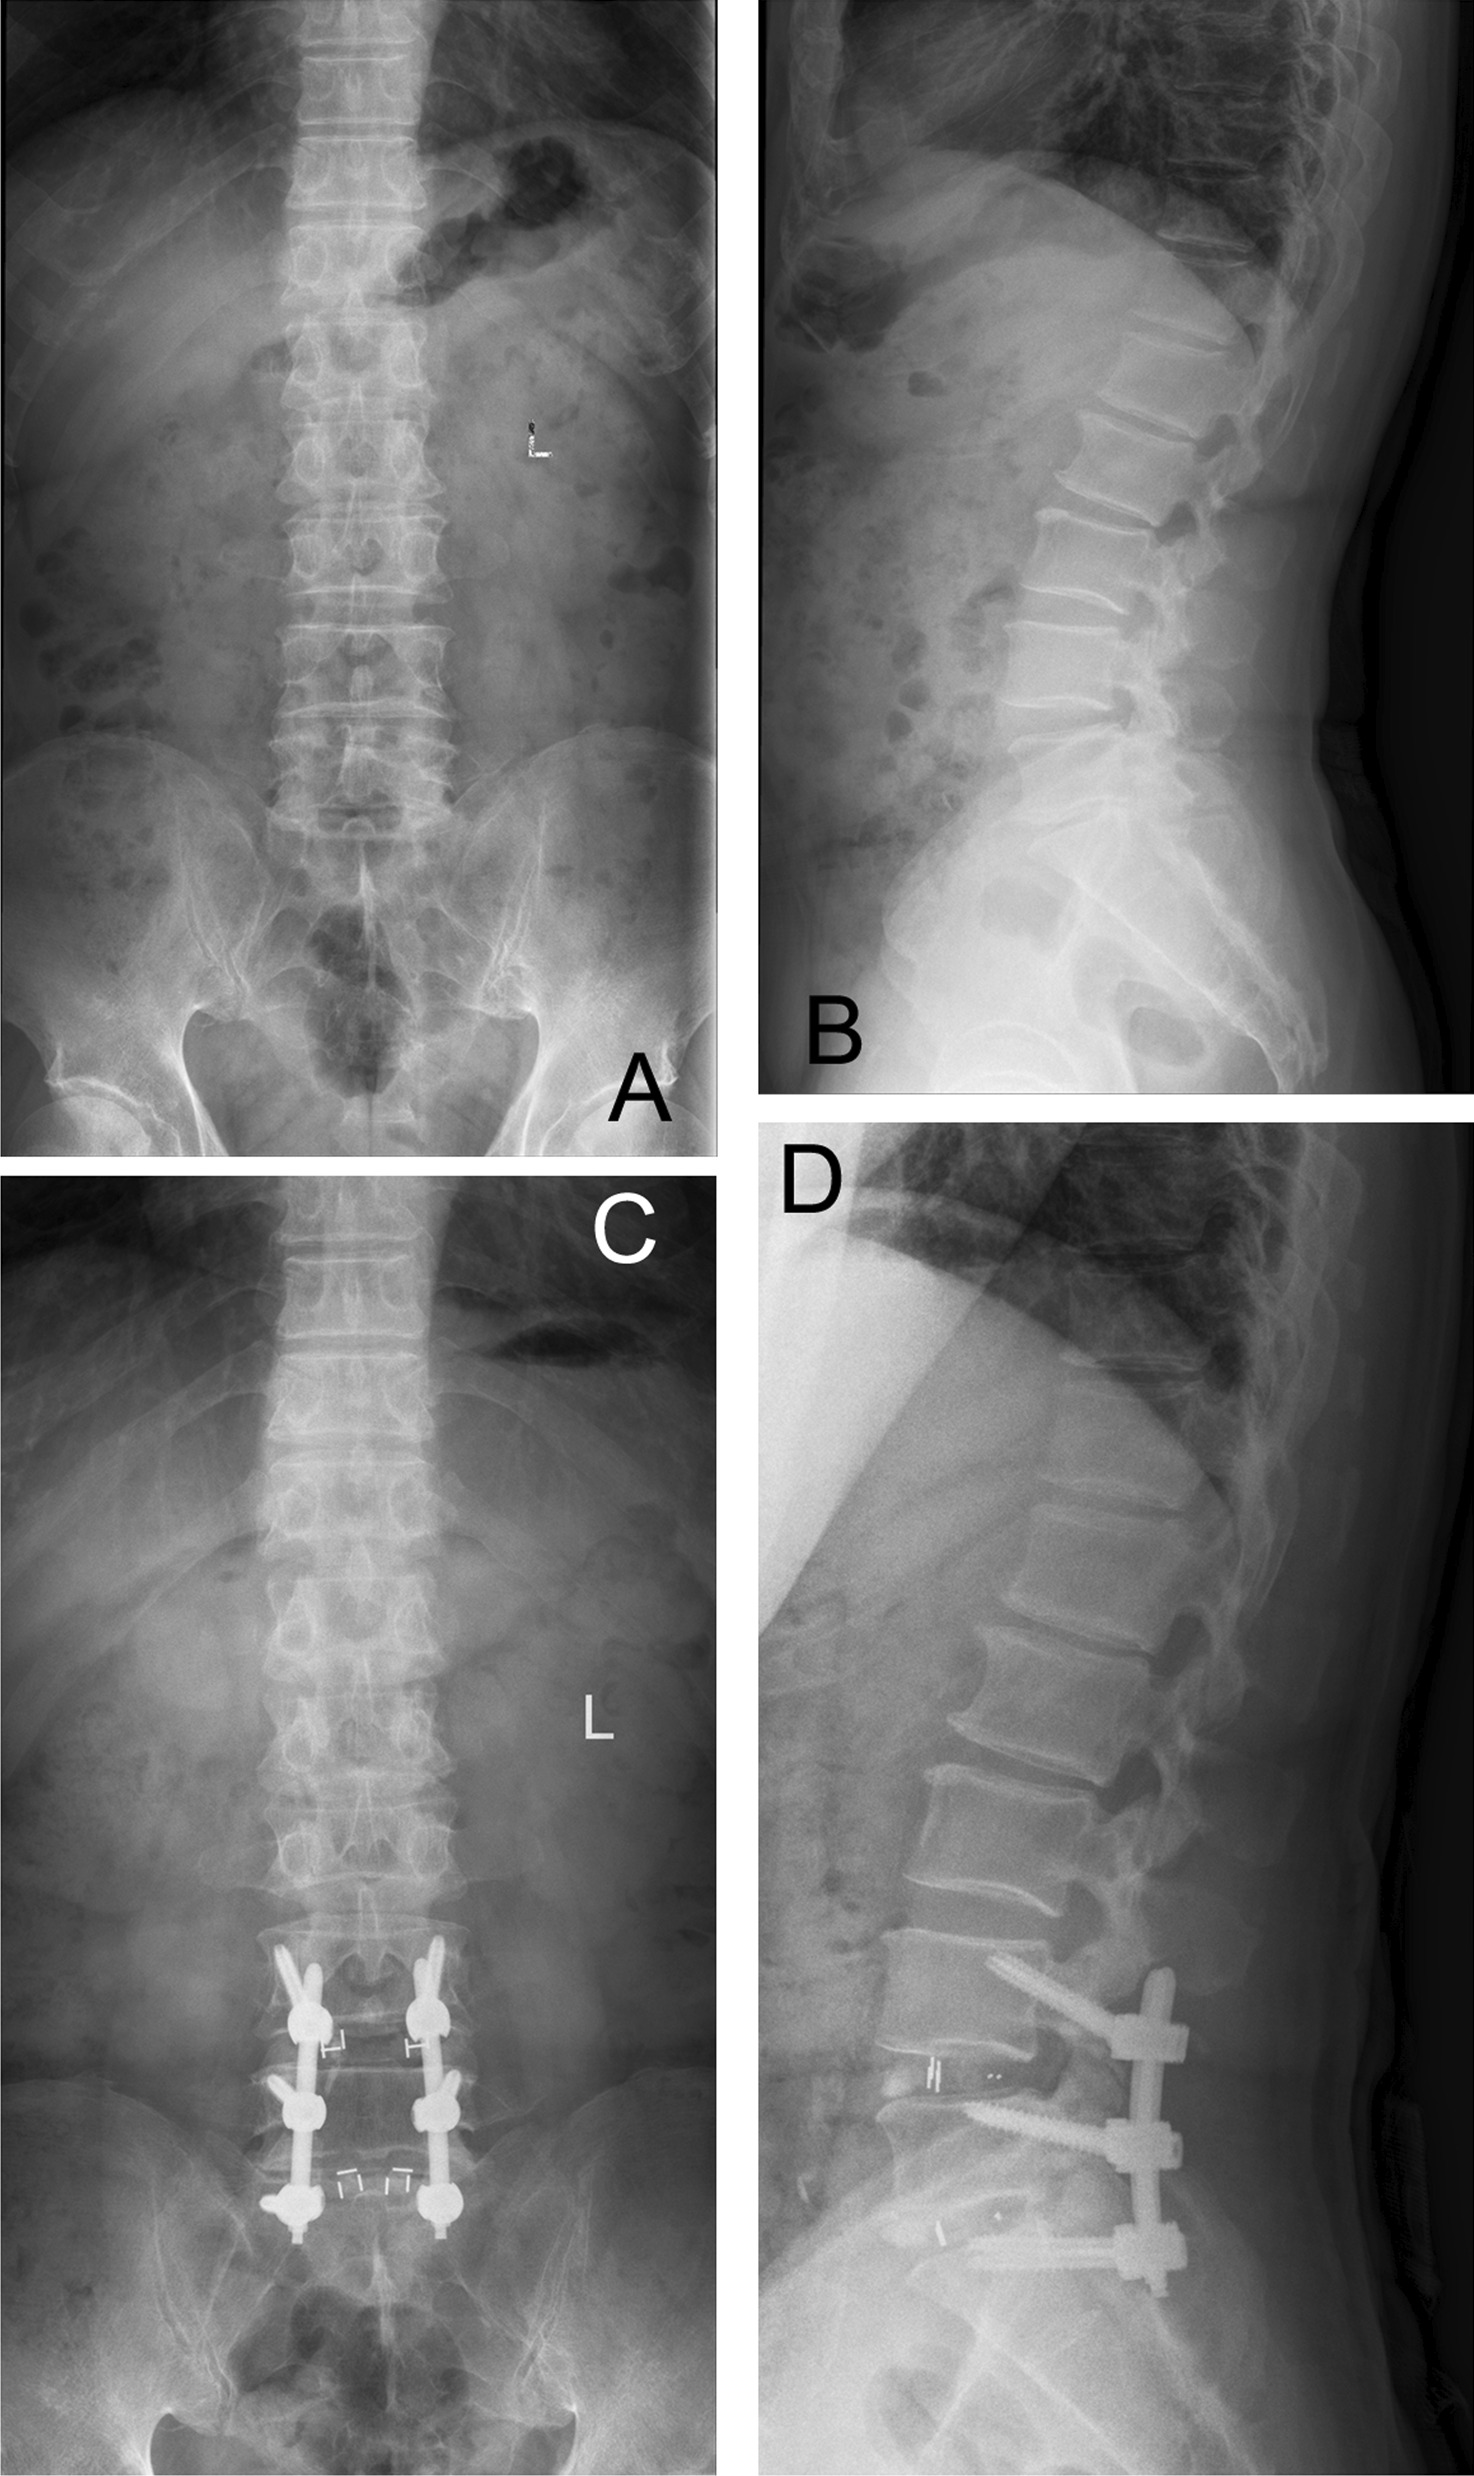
The preoperative and postoperative X-rays in a patient with lumbar spinal stenosis (L34, L45) underwent posterior laminar decompression, cortical bone trajectory internal fixation and interbody fusion. A The preoperative frontal X-ray. B The preoperative lateral X-ray. C The postoperative frontal X-ray. D The postoperative lateral X-ray
